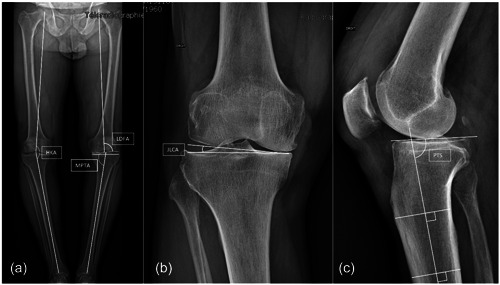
(a) Full‐length lower limb preoperative radiograph. Hip knee ankle angle was defined as the angle formed by the intersection of a line from the centre of the femoral head to the knee centre landmark and a second line from the centre of the ankle talus to the knee centre landmark. Lateral distal femoral angle was defined as the lateral angle between mechanical axis of the femur and the tangent line of the distal femoral condyles. Medial proximal tibial angle was defined as the medial angle between the mechanical axis of the tibia and the tangent line of the tibial plateaus. (b) Joint line convergence angle was defined as the lateral angle between the tangent line of the femoral condyles and the tangent line of the tibial plateaus. (c) Posterior tibial slope angle is formed between a line perpendicular to the longitudinal axis of the tibia and a line passing through the anterior and posterior high points of the tibial plateau.
